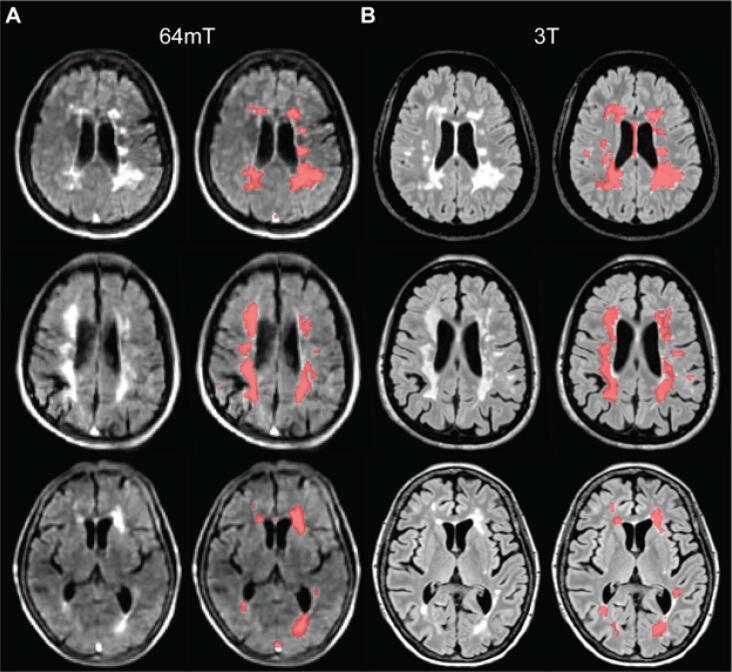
Automated lesion segmentations at 3T and 64mT overlap. (A) 64mT FLAIR images for three cases (left) with automated lesion segmentations generated from the 64mT images using MIMoSA overlaid (right). (B) Corresponding 3T FLAIR images for the same three cases (left) with 3T based segmentations (right). Patients from top to bottom are a 51-year-old female with RRMS, 44-year-old female with RRMS, and 71-year-old female with RRMS. All images were coregistered to 64mT T1-weighted images for comparison. Segmentations generated from 64mT and 3T scanners show similar patterns, although examples of false-positive segmentation in the sagittal sinus at 64mT can be seen in the top and bottom patients.
